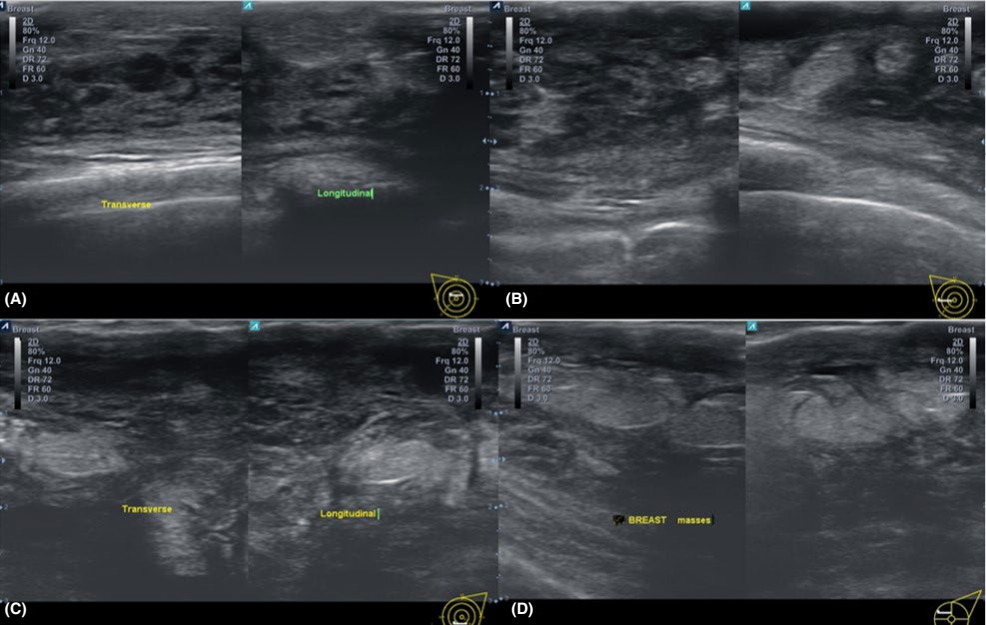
A, B, C, and D ultrasound of both breasts. A and B, Breast ultrasound of the right breast demonstrated multiple ill‐defined more hypoechoic lesions and less hyperechoic lesions at 12 O’clock above the nipple and at 9 O’clock, respectively. There were also subcutaneous lesions, skin thickening, and posterior enhancement. C and D—Breast ultrasound of the left breast demonstrated multiple ill‐defined more hyperechoic lesions and less hypoechoic lesions at 6 O’clock below the nipple and at 10 O’clock, respectively. There were subcutaneous involvement and skin thickening which are lesser than that of the right breast
